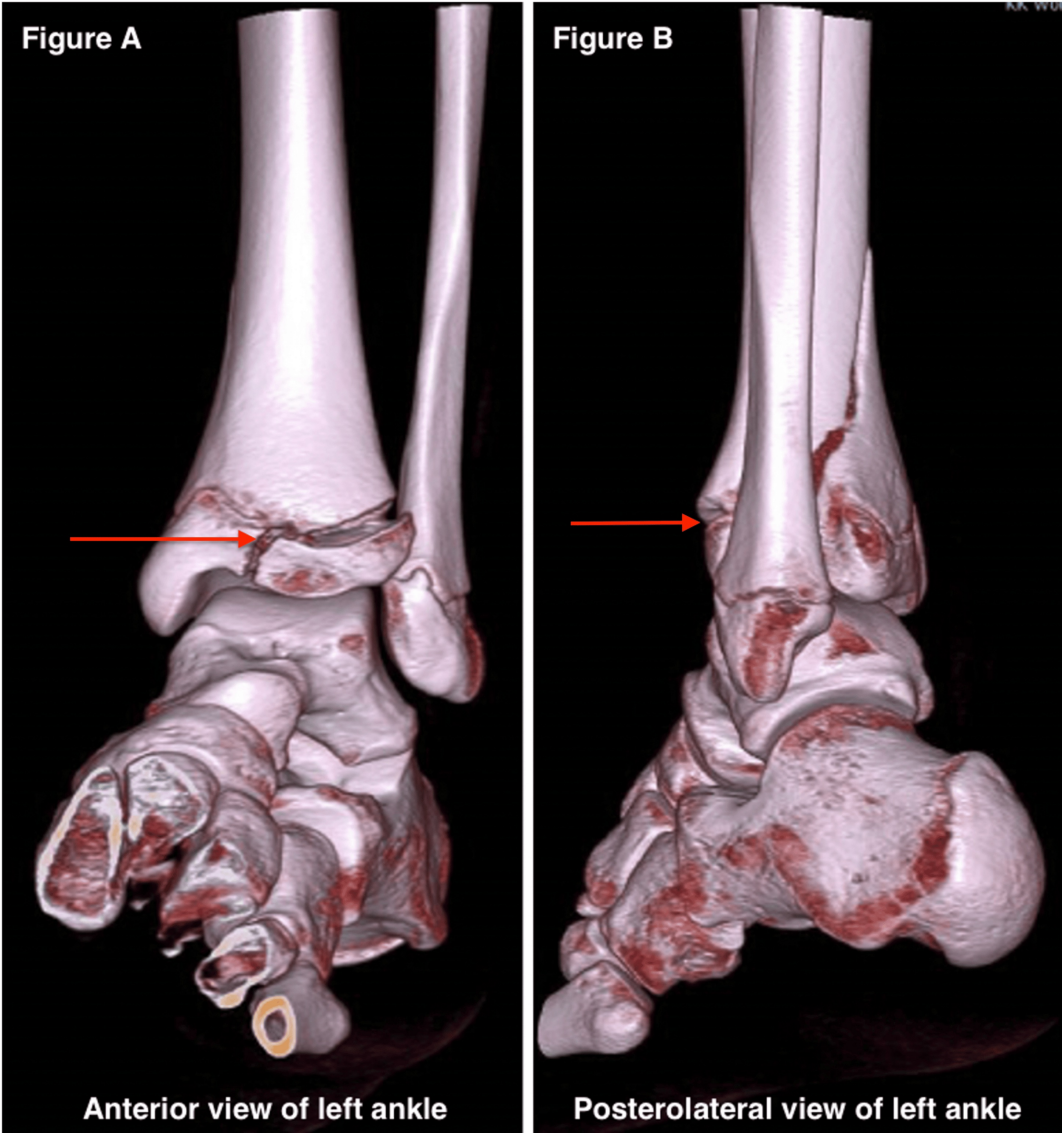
Preoperative computed tomography (CT) scan of a triplane fracture showing anterior (A, left) and posterolateral (B, right) views of the left foot. Arrows: Triplane fracture, a Salter-Harris type IV fracture at the distal tibia of the left ankle, involving metaphysis, physis (growth plate), and epiphysis.
